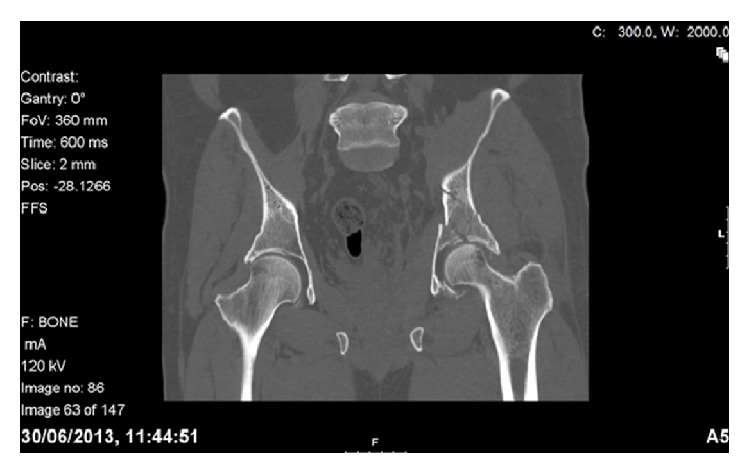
A coronal CT reconstruction suggesting medial subluxation of the femoral head.
